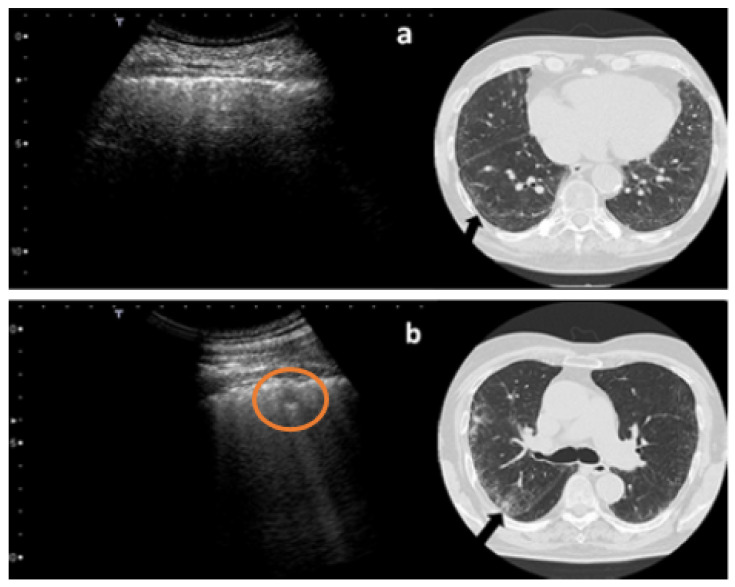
Ultrasound and CT scans of two patients with fibrosing lung disease. The black arrows indicate the position of the probe on the chest. The two CT and ultrasound scans are coplanar. In (a) a minor fibrotic involvement is present while in (b) a small nodulation (red circle) is evident.
